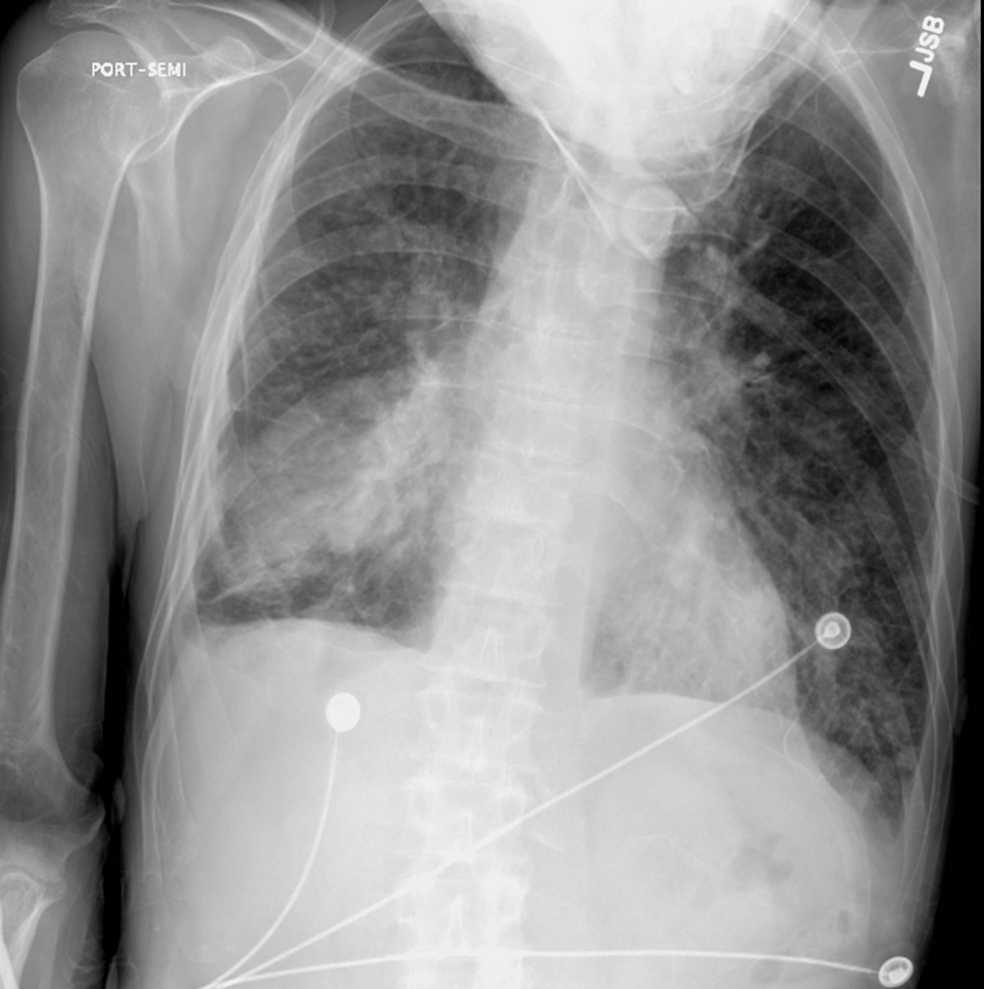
Chest X-ray showing right lower lobe airspace consolidation with interval development of findings suggestive of vascular congestion with congestive heart failure.
